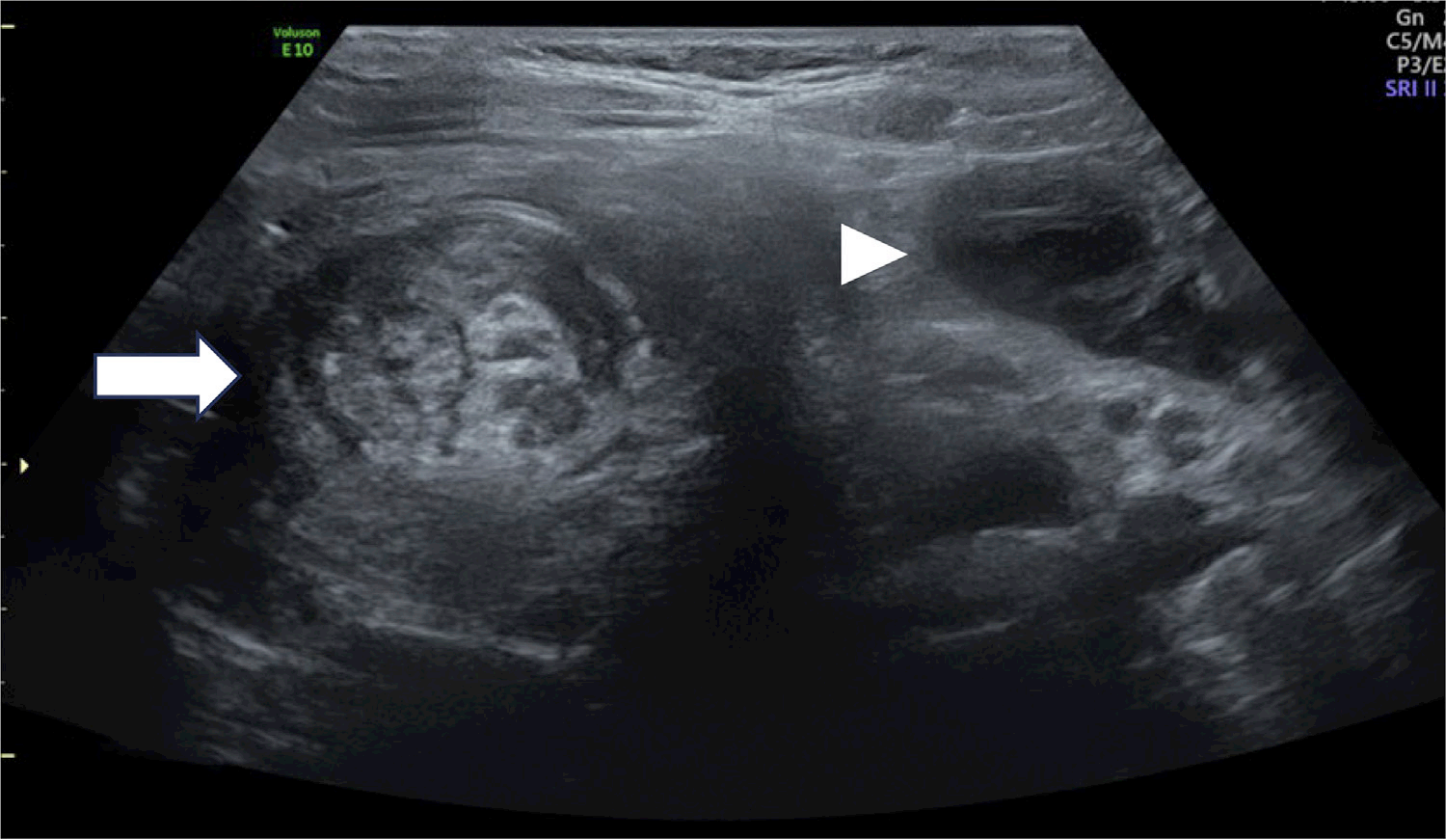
Transverse ultrasound image of the right iliac fossa showing the classic “*target*” appearance of ileocolic intussusception (white arrow), characterized by concentric hypoechoic and hyperechoic bowel wall layers. Adjacent to the intussusception, a well-defined cystic structure (white arrowhead) is visualized, suggestive of a cecal duplication cyst.
